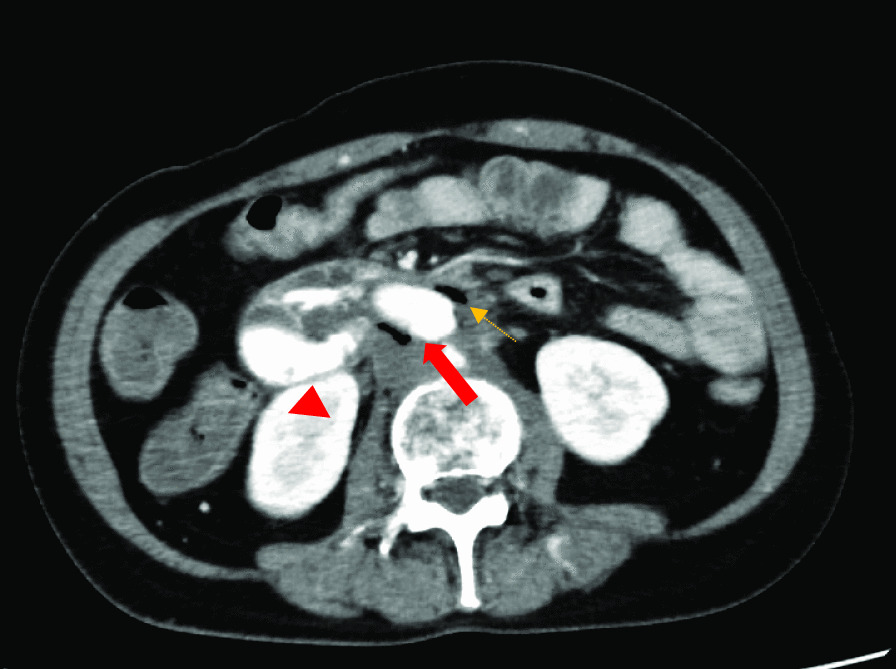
Contrast computed tomography image after an upper endoscopy (case 5). Arrow (solid red)/arrowhead (red): extravascular leakage from the aorta to the duodenum is observed. Arrow (dotted orange): image of air seen in the aorta
